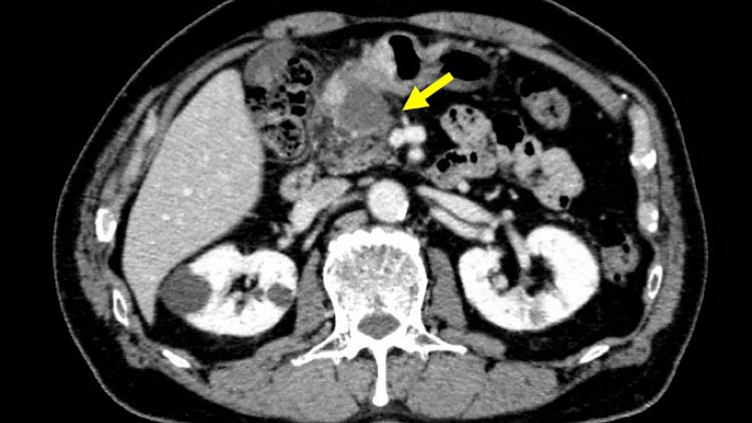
Abdominal CT showed the tumor at the gastric antrum visualized as a thickened wall, suggestive of direct invasion to the pancreatic head (arrow).
